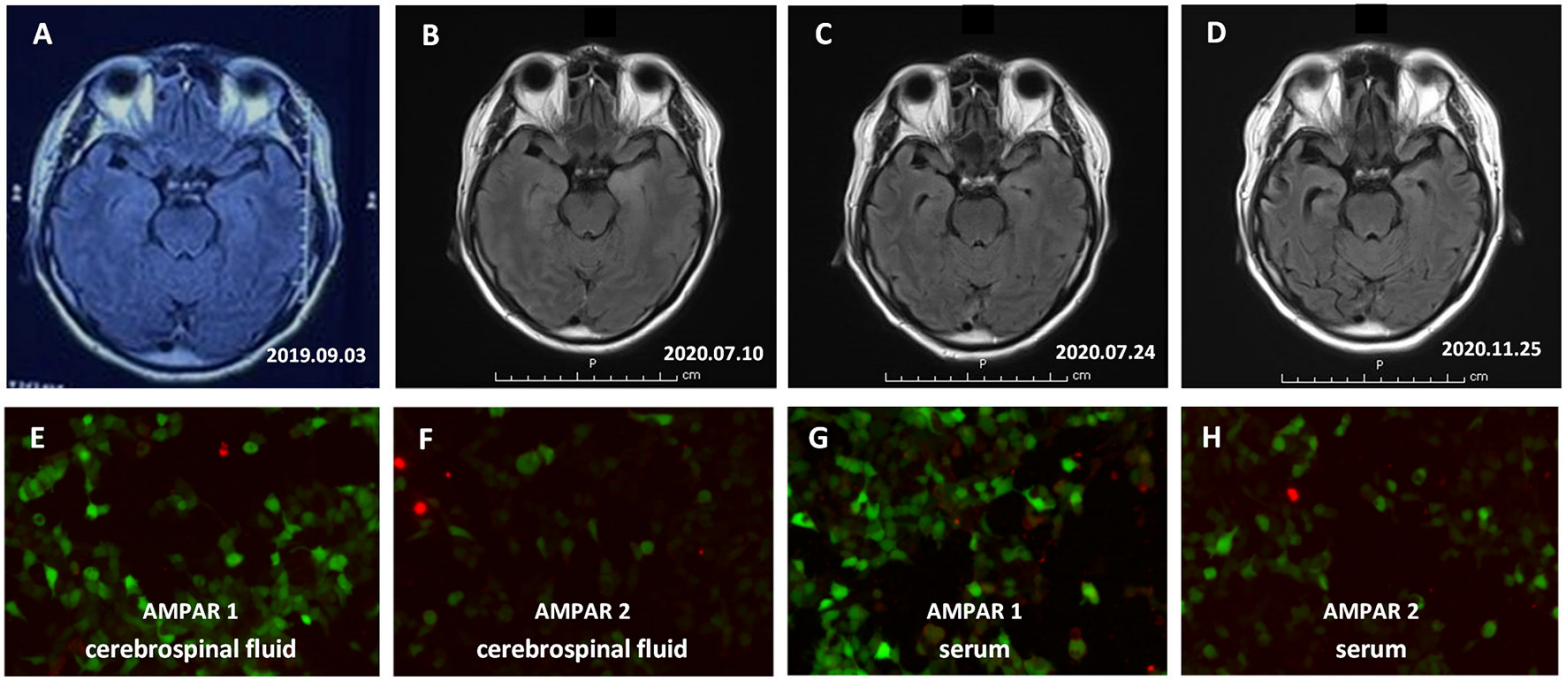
The result of Brain MRI and Anti-alpha-amino-3-hydroxy-5-methyl-4-isoxazolepropionic acid (AMPAR) antibodies in the serum and cerebrospinal fluid (CSF). (A–D) Brain MRI showed high signal changes on fluid-attenuated inversion recovery sequences predominantly affecting the bilateral medial temporal lobe combined with some parts of the temporal cortex and progressive brain atrophy. (A) At the onset of the disease; (B) Onset for more than 10 months; (C) After 2 weeks of hormone shock therapy; (D) Follow-up for 4 months after discharge. (E–H) Anti-AMPAR1 and Anti-AMPAR2 antibodies in the serum and CSF of the patient were positive, as tested by the CBA method. (E) Anti-AMPAR1 antibodies in CSF; (F) Anti-AMPAR2 antibodies in CSF; (G) Anti-AMPAR1 antibodies in serum; (H) Anti-AMPAR2 antibodies in serum.
